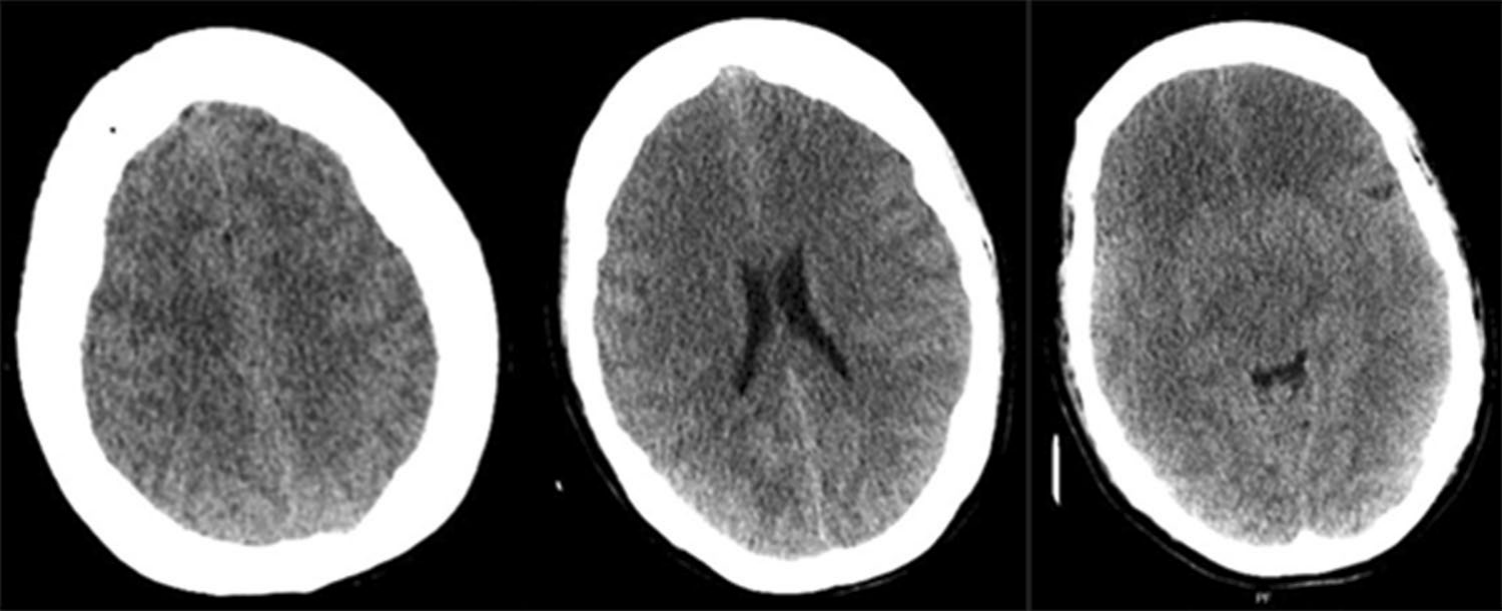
Initial CT scan showing general cerebral edema
